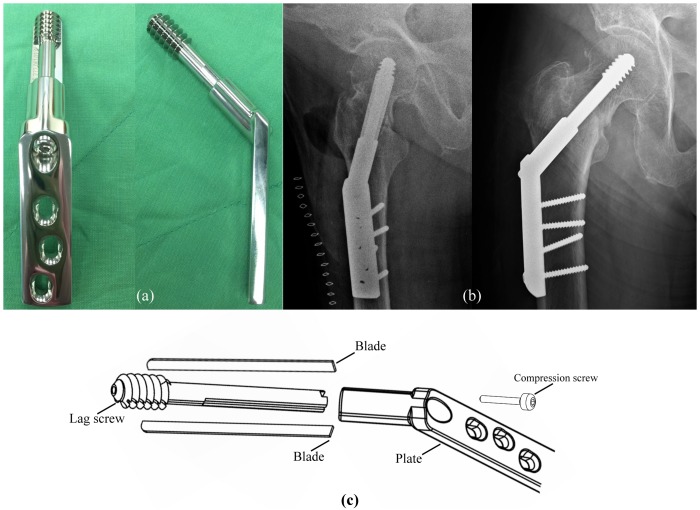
(a) Blade Screw DHS (ODRC Dynamic Hip Screw System, Chin Bone Corp., Taiwan; US FDA 510(k): K103015). (b) Radiographs of an 101 years-old woman showing fixation with a Blade Screw DHS post-operation. (c) The design and components of Blade Screw DHS.
